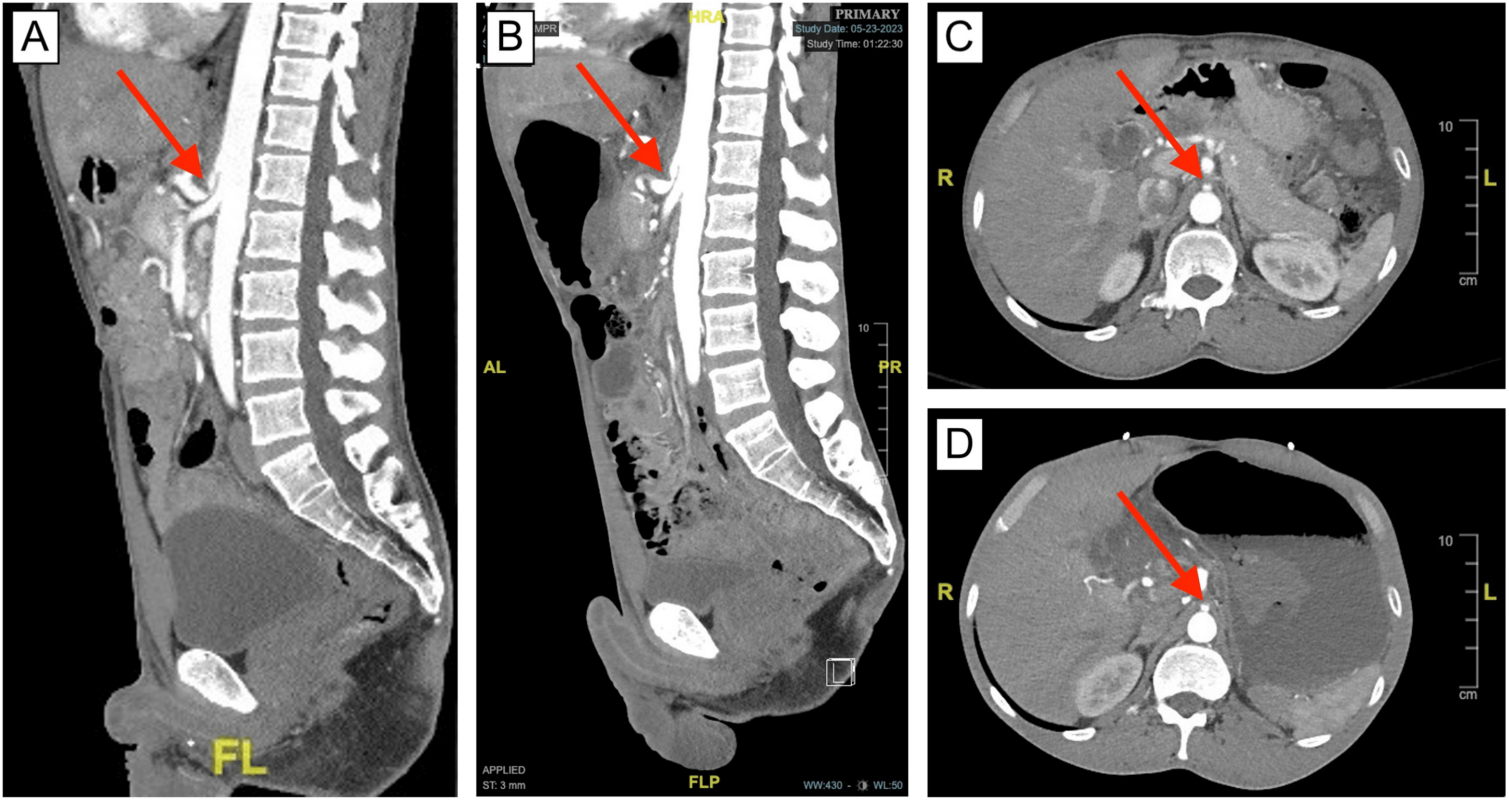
A CT angiography demonstrates compression of the celiac trunk in 2021 and 2023 (A/C) Sagittal and axial views of CT angiography taken in 2021 display the prototypical “J-hook” sign that is often associated with MALS and the location of the celiac trunk compression (red arrows). This medical encounter in 2021 was for a traumatic fracture of the right tibia and fibula, and the patient was not experiencing any GI symptoms at that time. (B/D) Sagittal and axial views of CT angiography taken in 2023 (time of relevant medical encounter) demonstrate celiac trunk compression (red arrows) imparted by the MAL. Post-stenotic dilation of the distal segment of the celiac artery can also be appreciated. There do not appear to be significant changes relative to the degree of celiac stenosis seen in 2021 (A/C) and 2023 (B/D).
